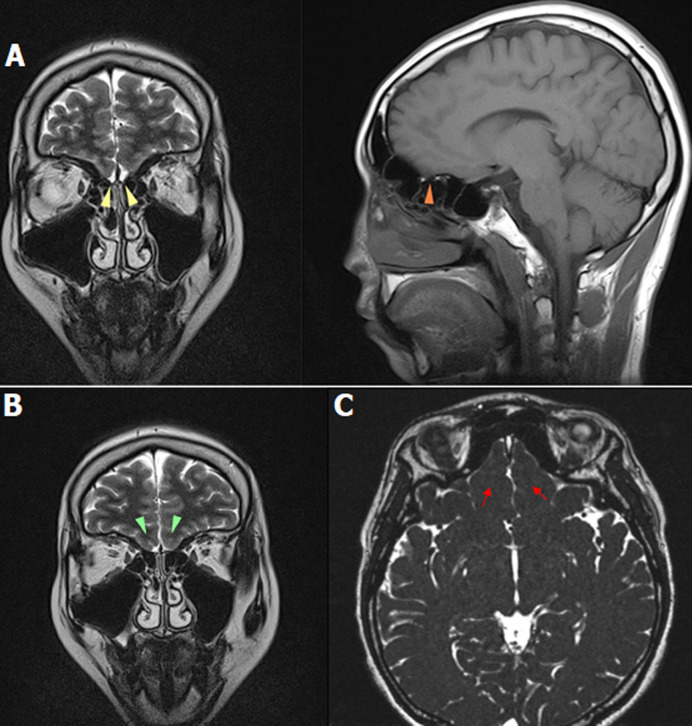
a coronal and sagittal T2-weighted MRI image showing bilateral olfactory bulbs agenesis (A), hypoplastic right olfactory sulcus, and aplastic left olfactory sulcus (B), an axial constructive interference in steady state (CISS) image showing an abnormal olfactory sulcus in an axial plane of CISS (C)
